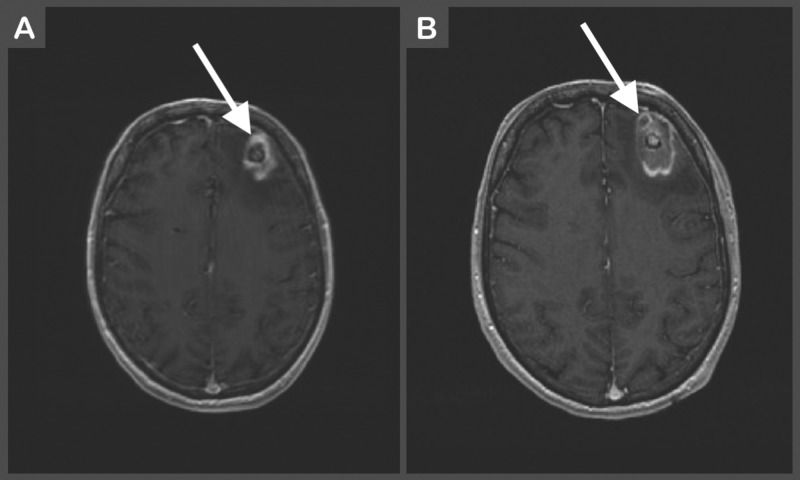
(A) Preoperative contrast-enhanced T1-weighted image of left frontal mass with surrounding vasogenic edema likely secondary to radiation necrosis. (B) Postoperative LITT contrast-enhanced T1-weighted image showing mixed-signal core intensity with thin peripheral enhancement consistent with expected complex hematoma. LITT: laser-induced thermal therapy
